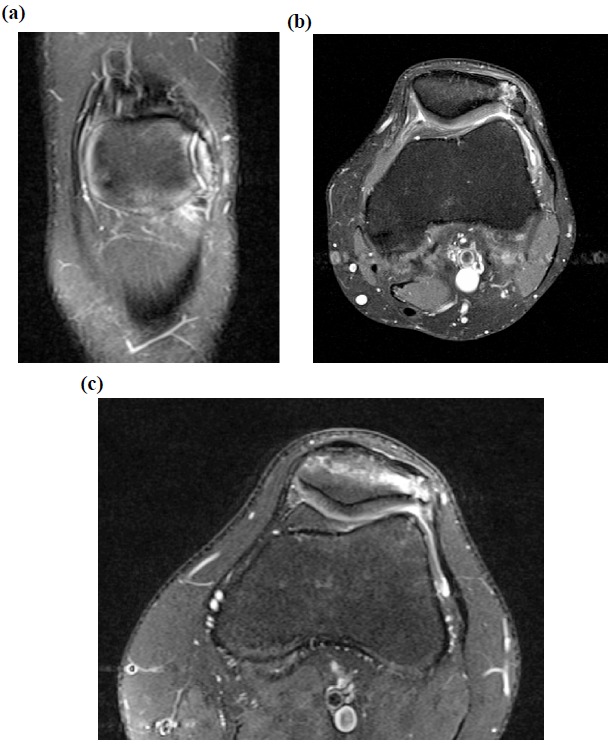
Pre-operative MRI images showing bipartite patella with disruption of the synchondrosis and a fissure in the underlying articular cartilage.
